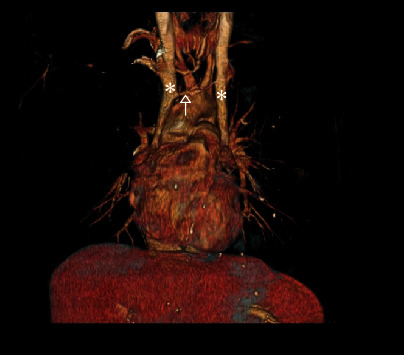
3D reconstruction view of the chest CT showing the two superior vena cava (white stars) with the communicating bridge passing anterior to the aortic arch (white arrow).
